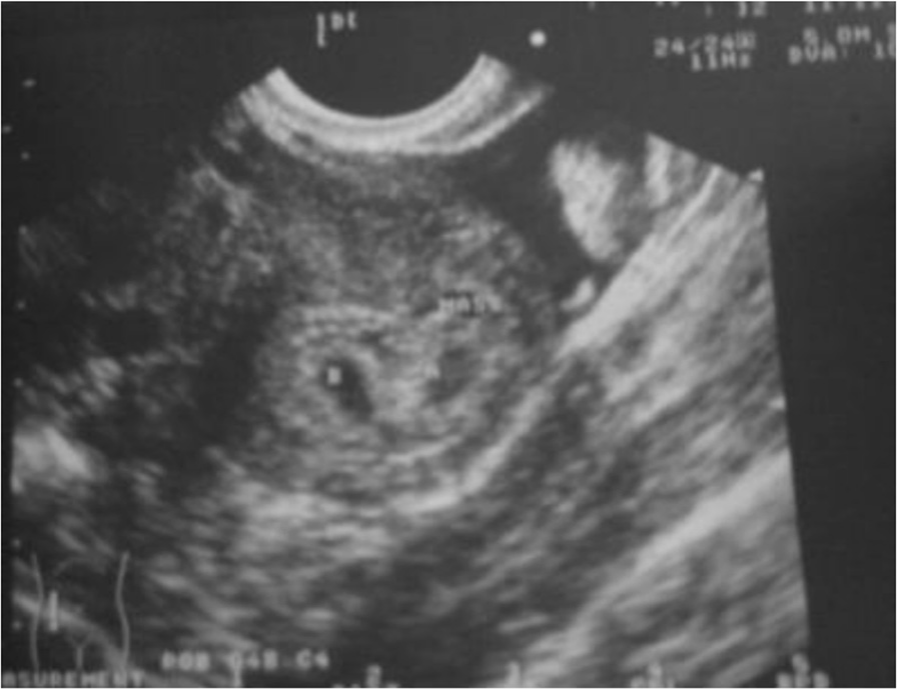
The transvaginal ultrasound revealed two different gestational sacs in uterine cavity and one had the fetal heart beat.
